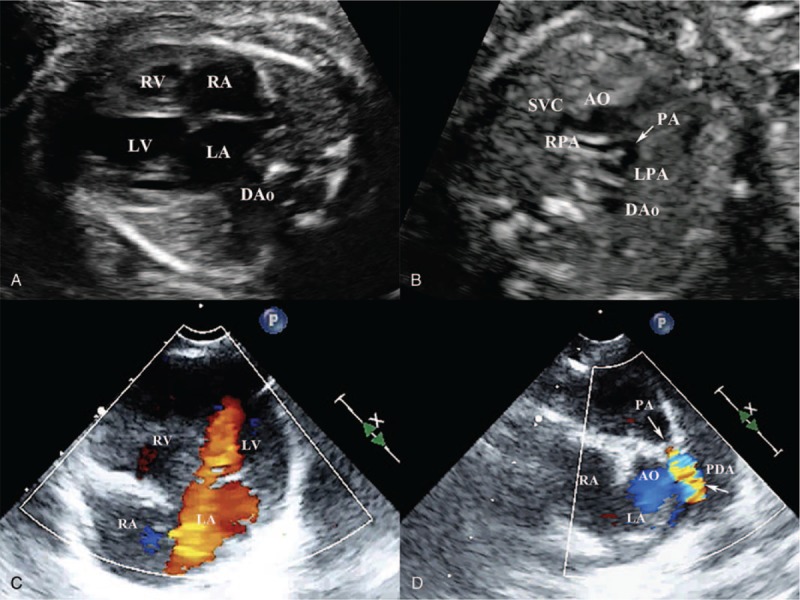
The prenatal and postnatal echocardiography of type II pulmonary atresia with intact ventricular septum. (A) The prenatal echocardiography at 22 weeks’ gestation, four-chamber view shows the absence of right ventricular trabecular portion, no venticulo-coronary artery communication, intact ventricular septum. (B) The 3-vessel-trachea view shows pulmonary atresia (*narrow*). (C) The postnatal echocardiography of the same fetus, four-chamber view shows the absence of right ventricular trabecular portion, no venticulo-coronary artery communication, no tricuspid regurgitation. (D) Parasternal short axis shows pulmonary atresia (*arrow*), patent ductus arteriosus. AO = aorta, DAo = descending aorta, LA = left atrium, LPA = left pulmonary artery, LV = left ventricle, PA = pulmonary atresia, PDA = patent ductus arteriosus, RA = right atrium, RPA = right pulmonary artery, RV = right ventricle, SVC = superior vena cava, VCAC = ventriculo-coronary artery communication.
